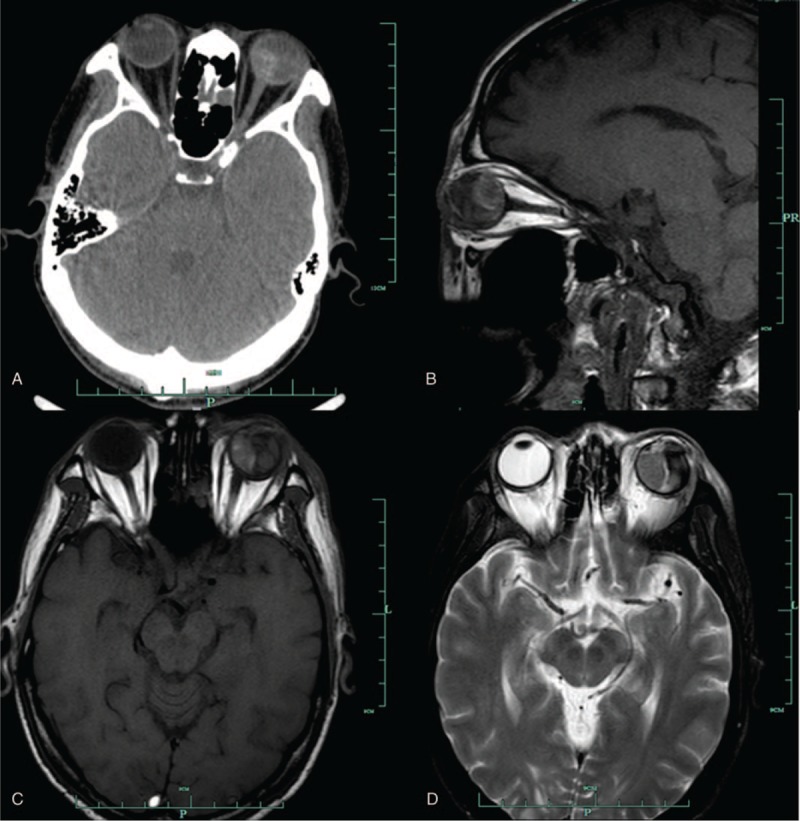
(A) Computed tomography scan showing an enhanced mass at the nasal and posterior side of the left eye; (B) MRI scan showing the same lesion on the left eye; (C) The T1-weighted image shows isointensity with enhancement; (D) The T2-weighted image shows hypointensity. MRI = magnetic resonance imaging.
